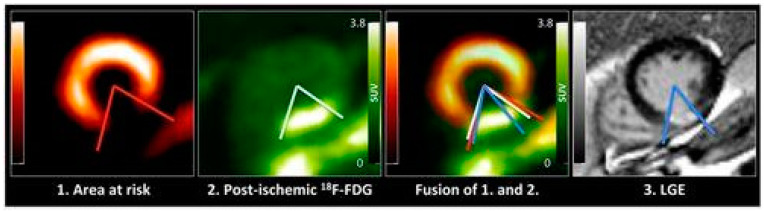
Increased myocardial FDG uptake in the postischemic myocardium reflects the inflammatory response. Early after myocardial infarction, there is an intense ^18^F-FDG uptake in the myocardium. The regional analysis of cardiac ^18^F-FDG and LGE extent confirmed that the ^18^F-FDG extent significantly exceeds the LGE extent. The area at risk depicted by ^99m^Tc-sestamibi SPECT matched roughly the post-ischemic ^18^F-FDG uptake [4].
